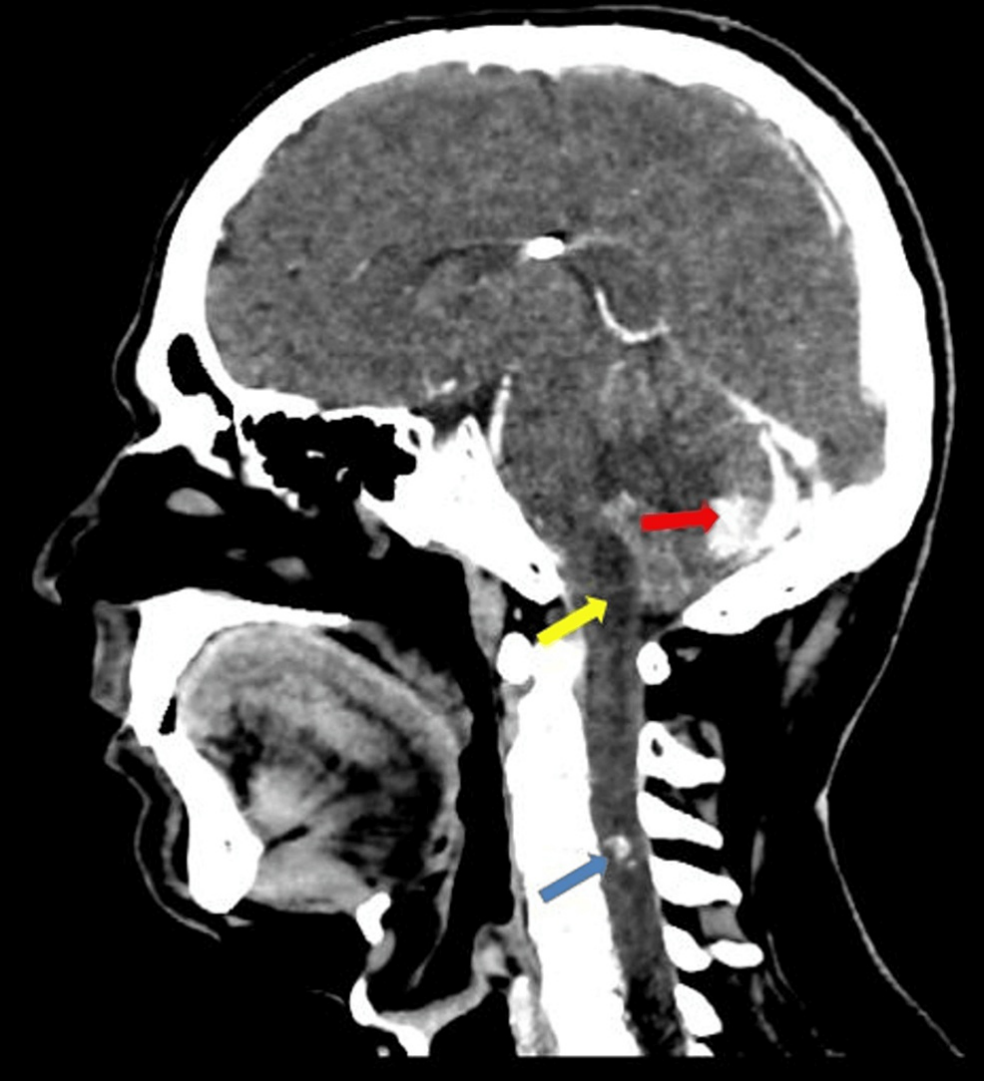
Sagittal reformatted contrast-enhanced computed tomography image This figure shows an enhancing lesion in the cervical spinal cord (blue arrow), with the central canal of the spinal cord above exhibiting hydromelia with a syrinx (yellow arrow), and an enhancing lesion in the left cerebellar hemisphere (red arrow)
